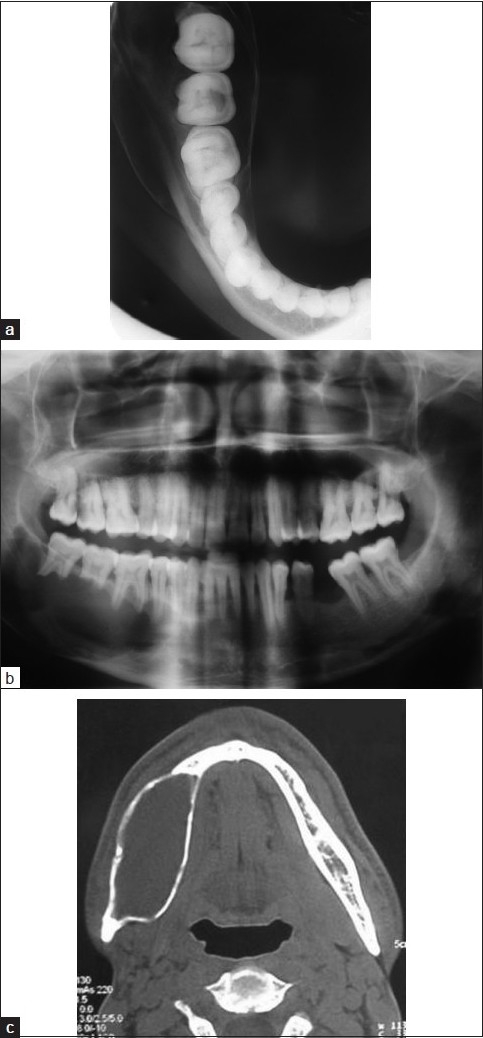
(a) Mandibular occlusal radiograph showing expansion of the cortical plates; (b) panoramic radiography showing a unilocular radiolucency extending from 45 to 48 region; (c) axial CT image of the mandible showing cortical expansion and thinning
